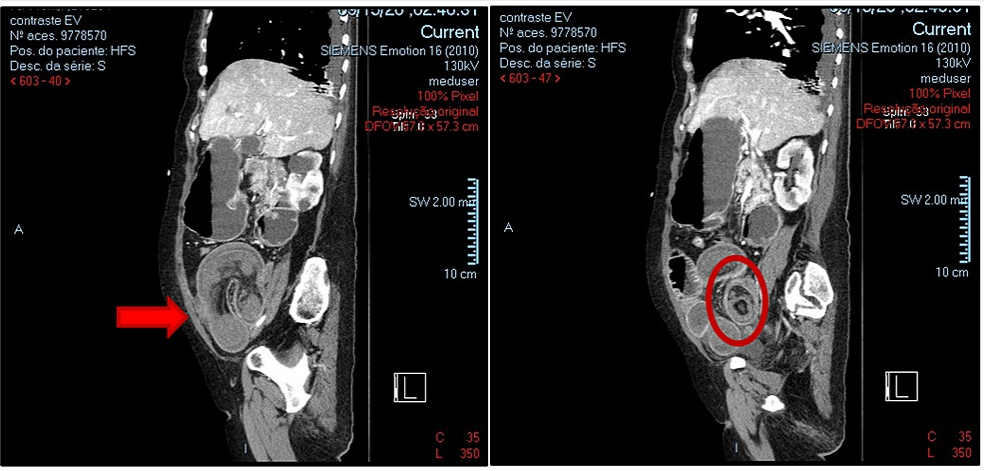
CT scan with typical findings of bowel inside bowel, suggesting intussusception (sagittal views) with special note to the target sign appearance on the image on the right
